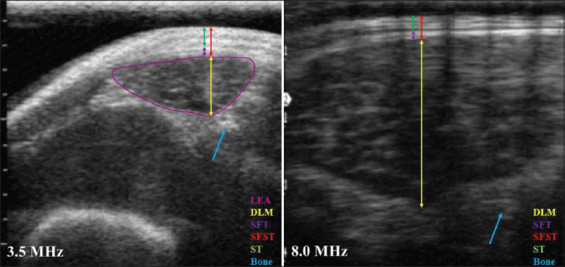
Ultrasound image obtained with 3.5 or 8.0 MHz frequency. Indicating measurements of loin eye area, *Longissimus thoracis et lumborum* muscle depth, subcutaneous fat thickness, subcutaneous fat plus skin thickness, skin thickness.
